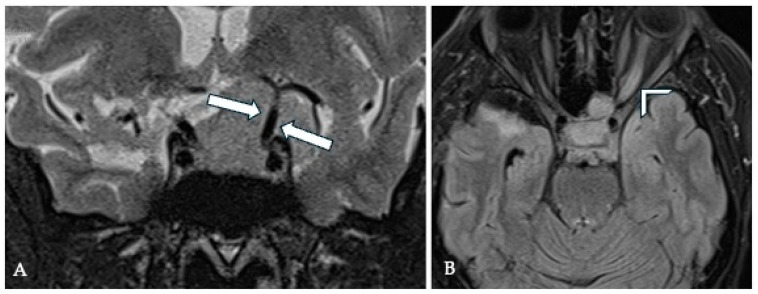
Coronal fine-section T2WI of a patient with a history of TSM resection via right pterional craniotomy at another center, now presenting with left eye vision loss (A). Imaging reveals complete encasement of the left ICA and bilateral cavernous sinus involvement (CSI). Hyperintensity surrounding the left ICA indicates the presence of an arachnoid cleavage plane, with no evidence of vessel narrowing (arrow). Axial FLAIR imaging (B) demonstrates marked edema of the left optic nerve (ON), correlating with the patient’s preoperative visual impairment (arrowhead).
